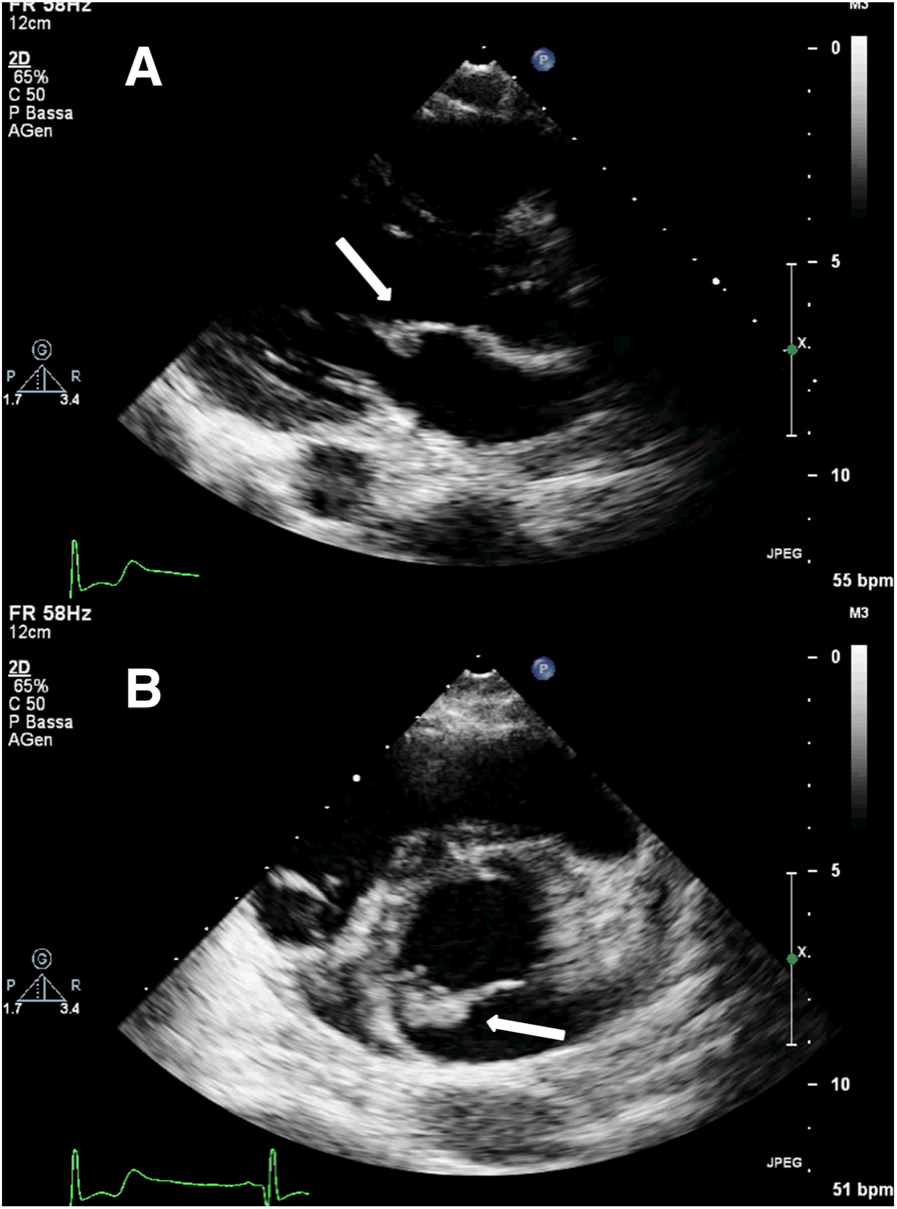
Transthoracic echocardiogram. The long axis (panel a) and short axis views (panel b) show the hyperechogenous mass (*white arrow*) attached to atrial side of A3 scallop of the anterior mitral leaflet
